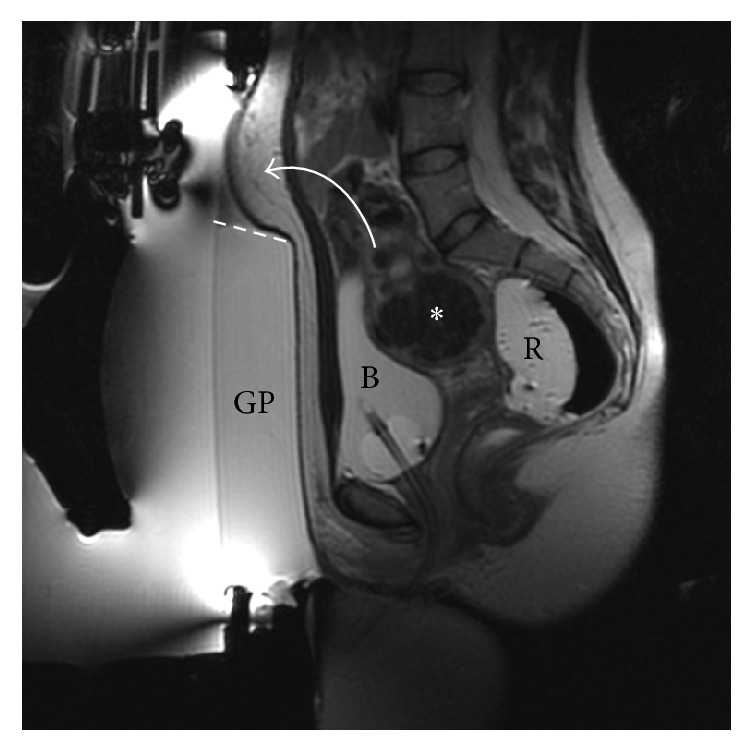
Case 2: sagittal T2-w intraoperative scan showing the targeted fibroid (asterisk) and full bladder (B) with urinary catheter present. Bowels obstructing the beam path were mitigated using a custom sliced (dashed line) gel pad (GP) and rectal filling with ultrasound gel (R). Curved arrow mimics the dislocation of the bowel loops. This treatment was successfully performed through the full bladder.
